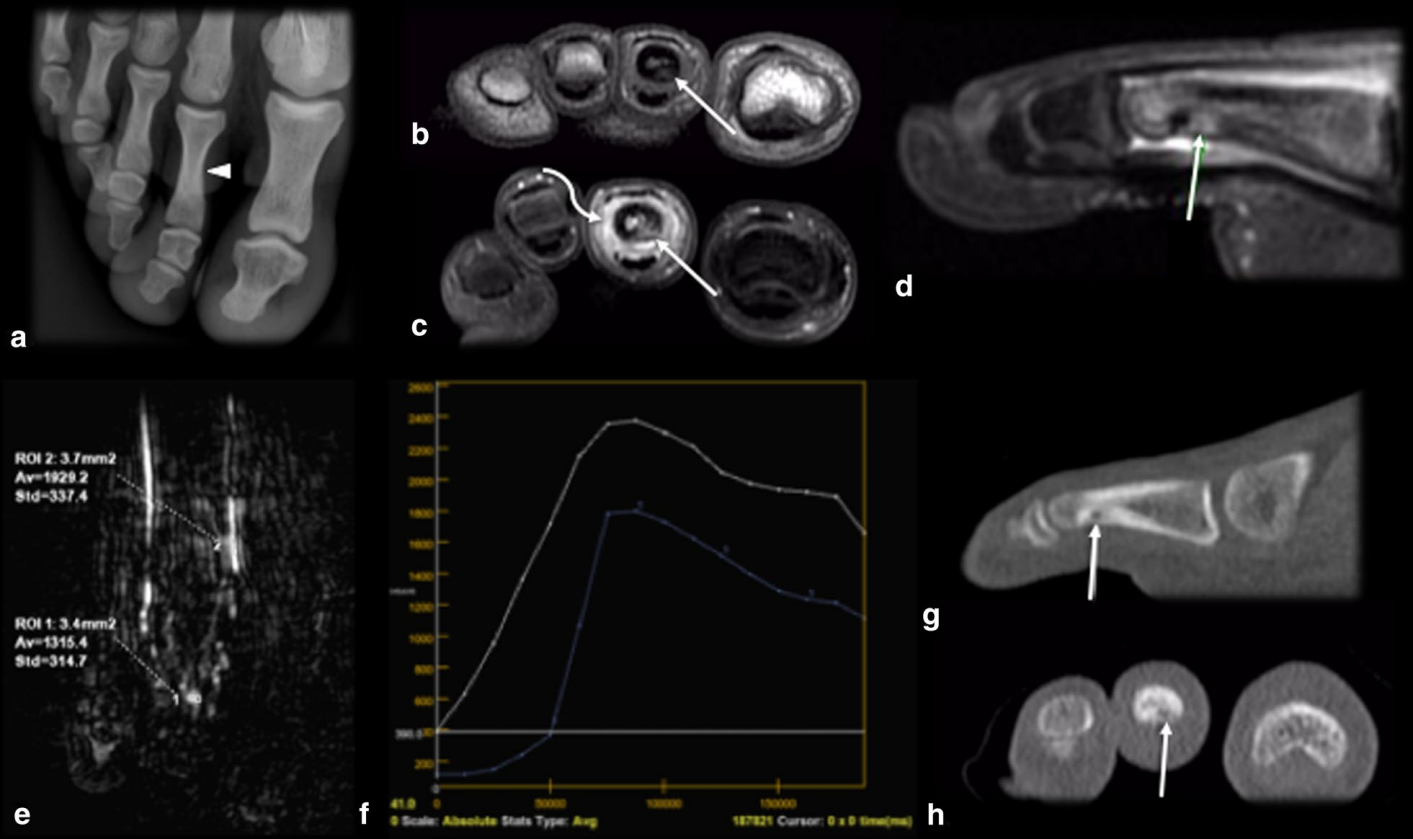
Pattern of enhancement of osteoid osteoma. Male, 17 years old, presenting with metatarsalgia of the right foot for 2 months, which became worse over the past week. He had no recollection of trauma and practiced sports regularly. CR (a) showed no significant findings, apart from mild bone sclerosis in the middle phalanx of the second toe (arrowhead). Coronal T1 and T2WI (b, c) depicted marked edema of the bone marrow and surrounding tissues (curved arrow) and a very small intracortical lesion (dashed arrow). Dynamic MR angiography (d–f) showed marked enhancement of the intracortical nodule (arrow in d), presenting contrast kinetics similar to those of adjacent arteries, with a peak enhancement followed by rapid washout, suggestive of OO (e, f). A CT scan (g, h) was later performed, and the findings confirmed the presence of a nidus (arrows)
